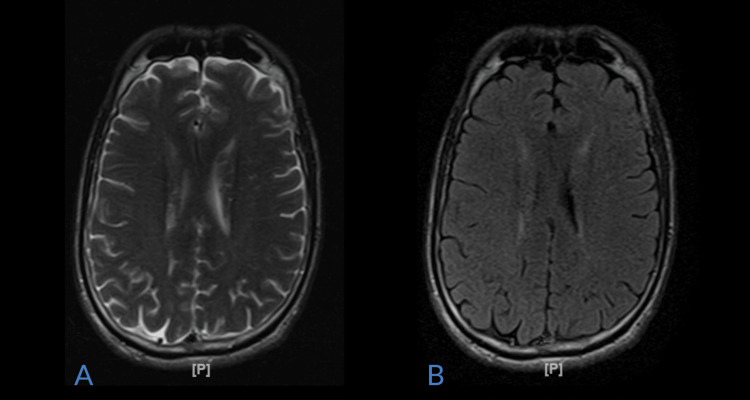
T2 axial (A) and axial flare (B) magnetic resonance imaging demonstrating no vasogenic edema in the parieto-occipital regions on the third day of hospitalization
